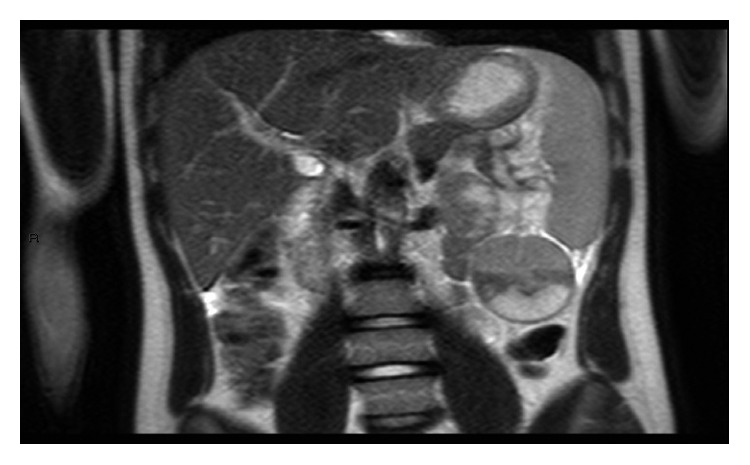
T2-weighted gadolinium-enhanced coronal view of MRI showing hemorrhage within the AS.
